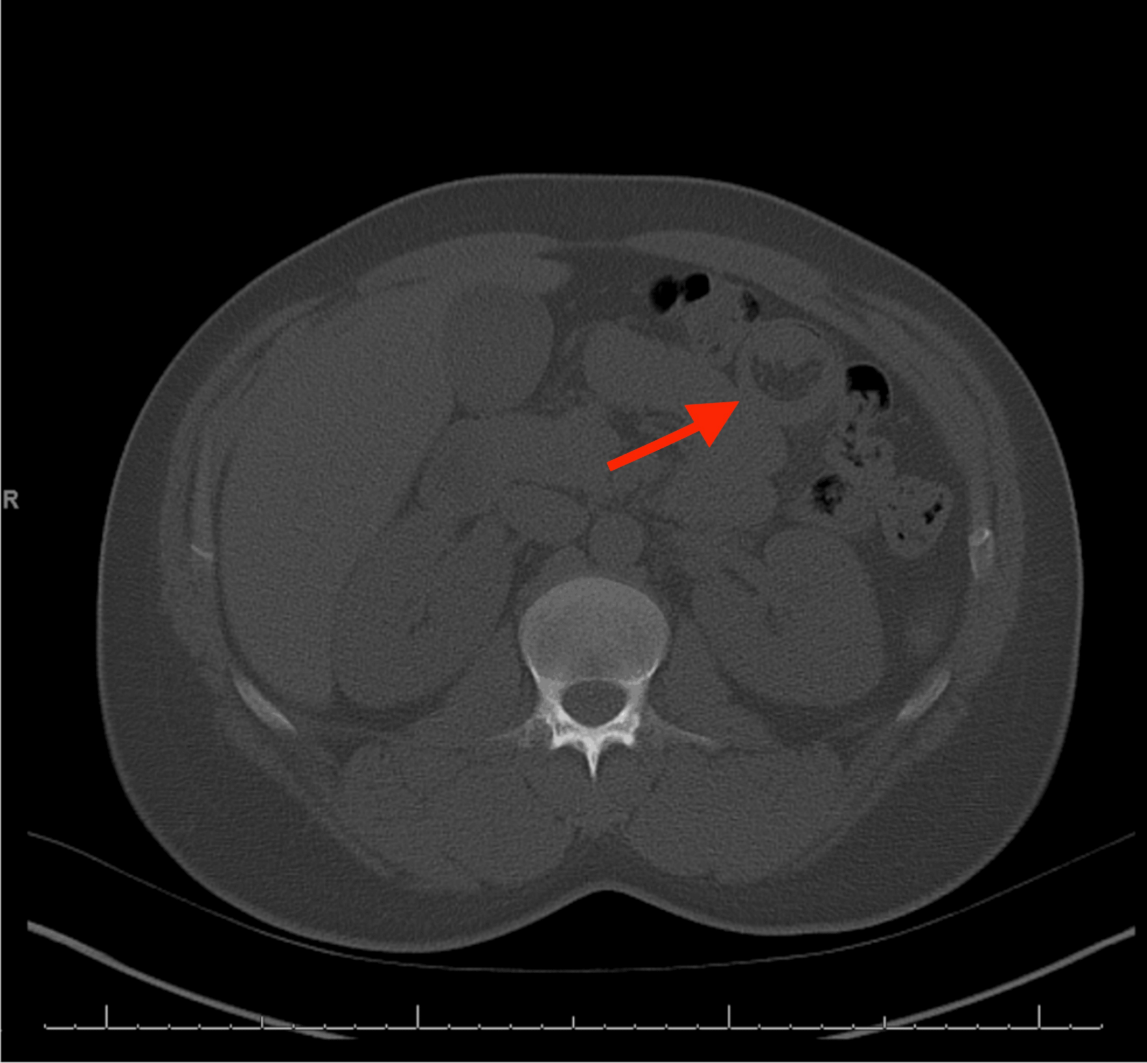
Axial CT scan of the abdomen without contrast demonstrating a 'target' appearance consistent with enteroenteric intussusception (red arrow) No evidence of obstruction, mass lesion, or ischemic changes was observed.
